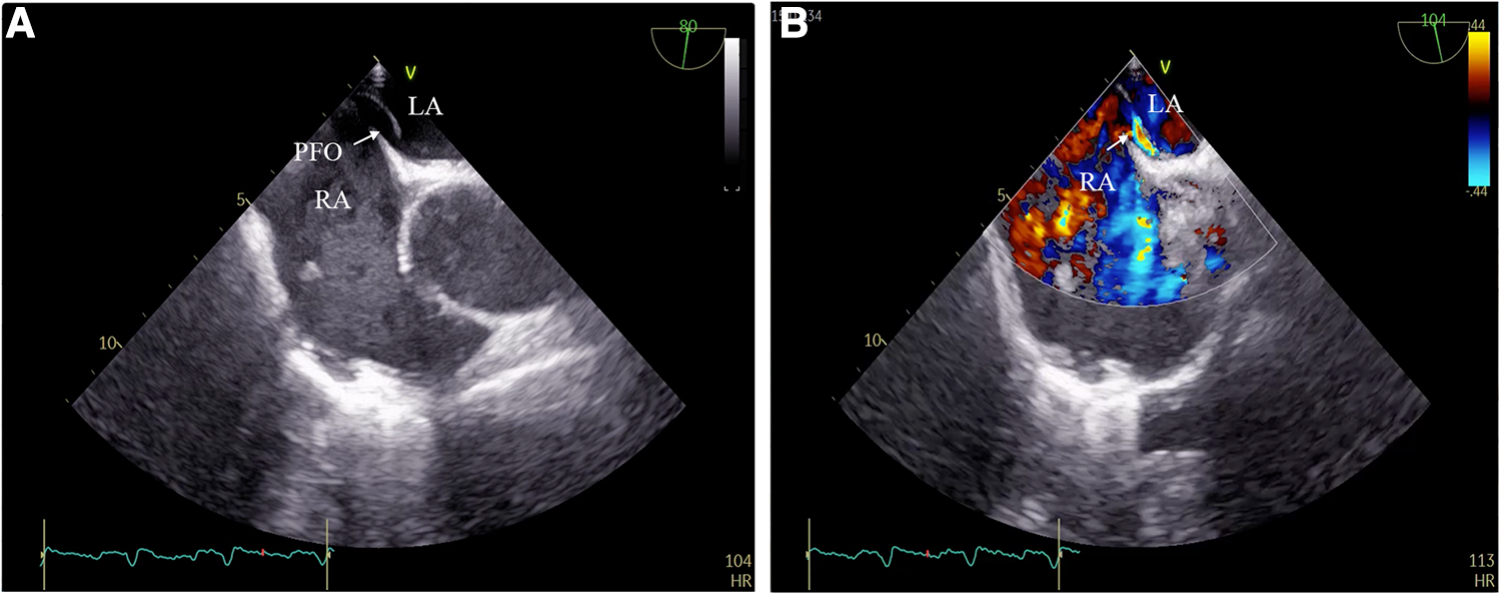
(A) PFO detected by transesophageal echocardiography; (B) right-to-left intracardiac shunt through PFO.
